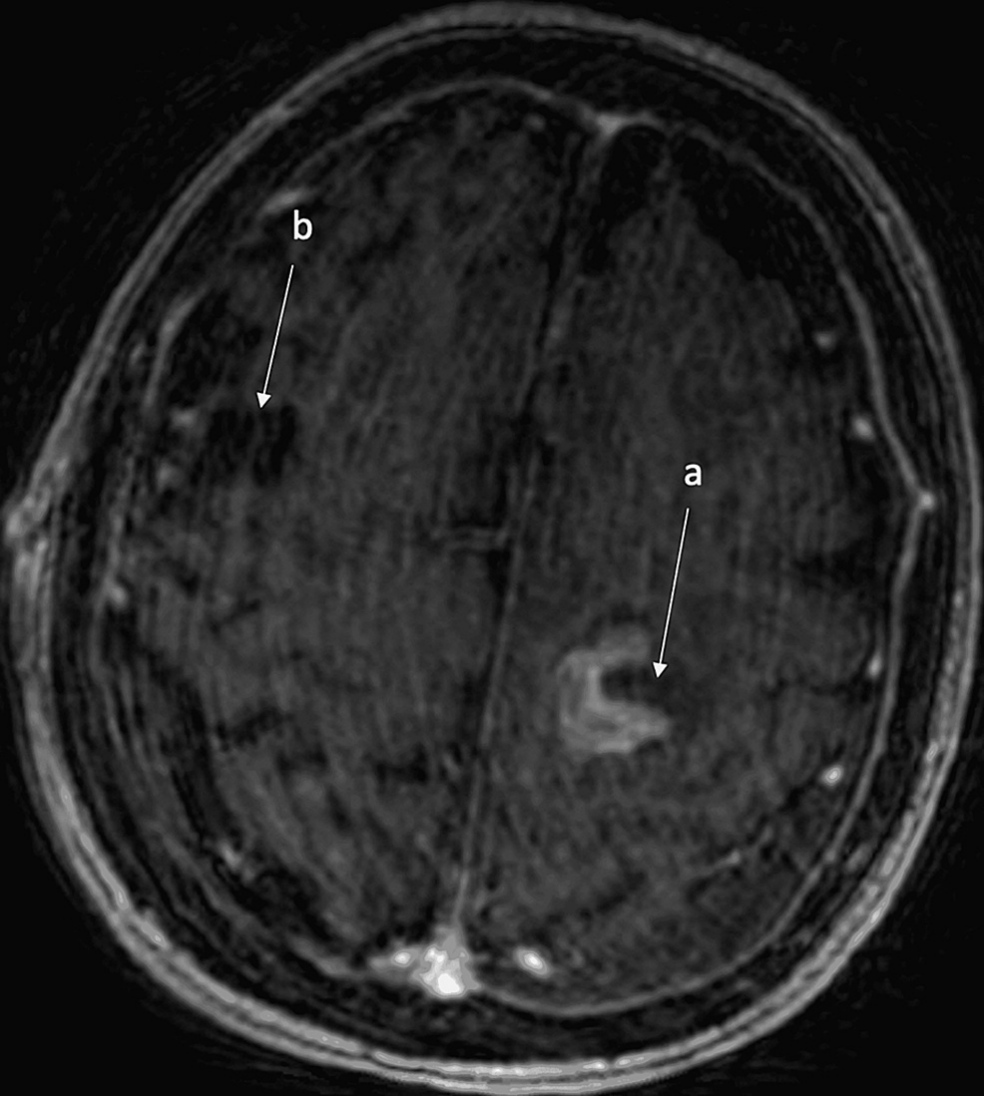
Motion degraded post-operative image demonstrates interval resection of the left frontoparietal (a) and right posterior frontal lesions (b) with a small amount of blood involving the surgical resection (left > right) without evidence of enhancement and a small left posterior convexity subdural hematoma. MRI - magnetic resonance imaging.
